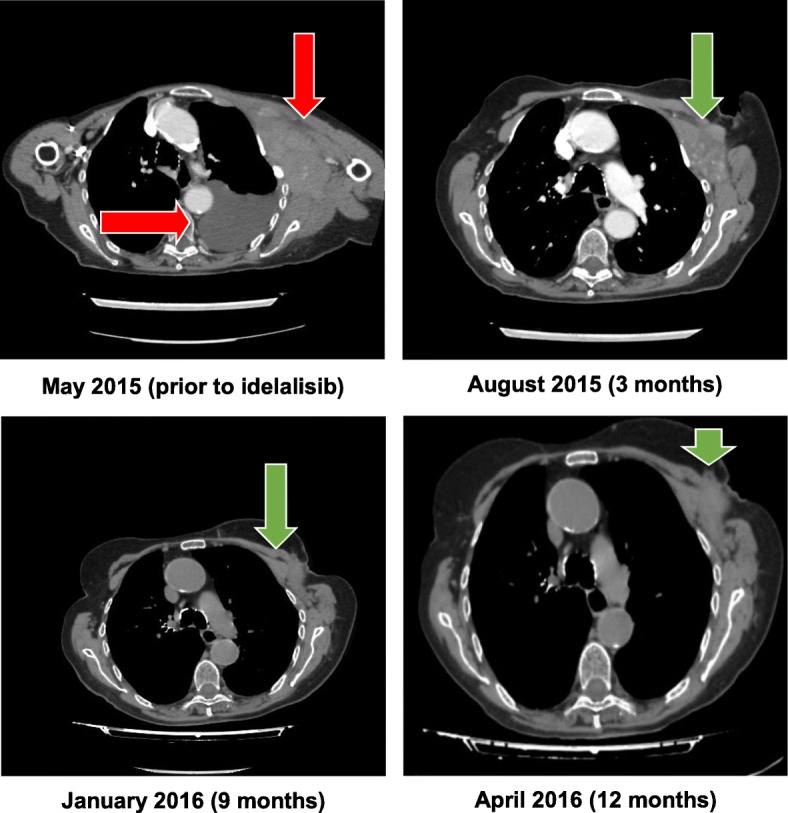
Computed tomography response to idelalisib treatment. Arrows indicate presence of lymphoedema and/or pleural effusion
